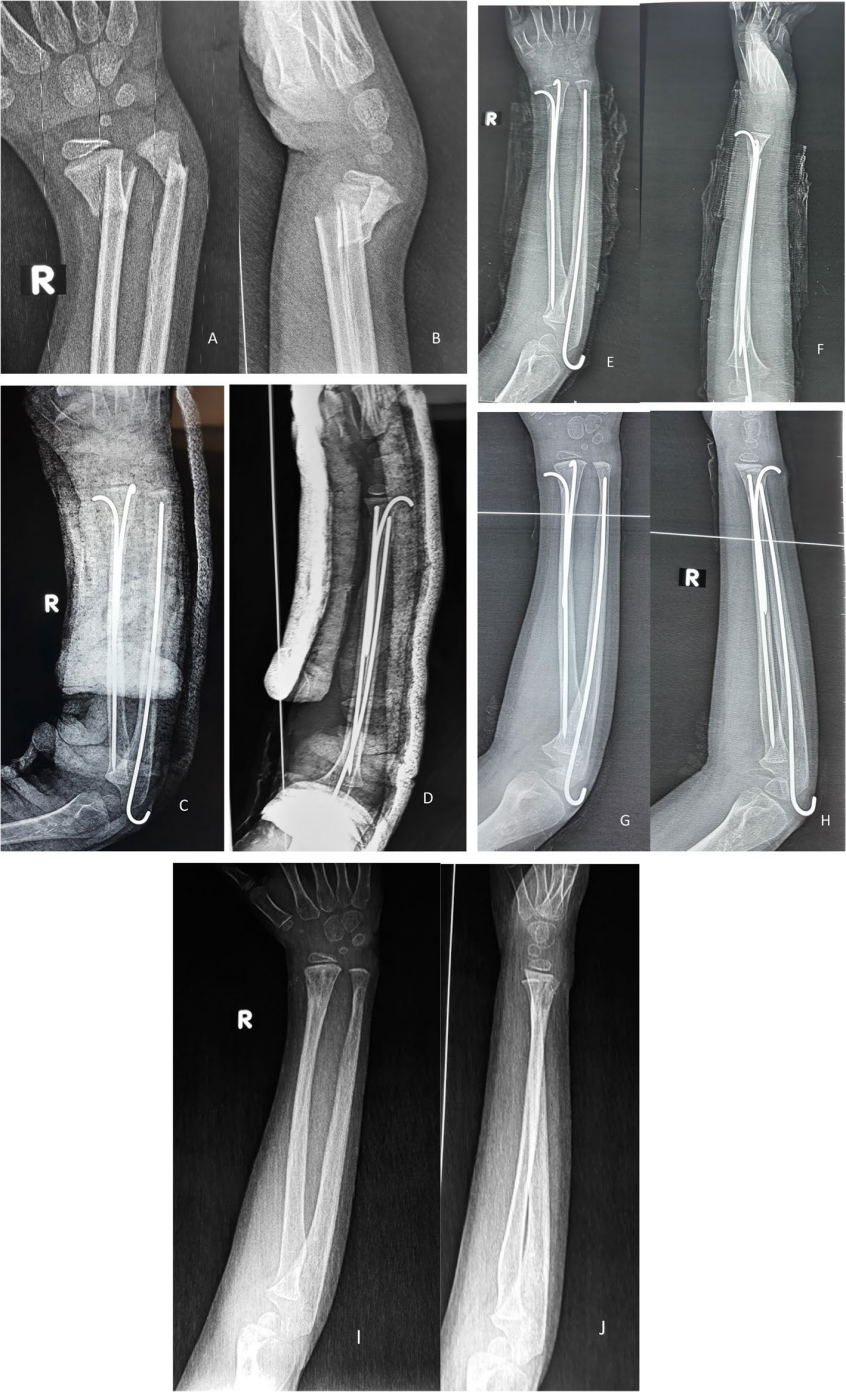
A and B. Anteroposterior and lateral X-rays show a distal forearm fracture in a male 7-year-old patient. C and D. Anteroposterior and lateral X-rays show the immediate postoperative radiograph. E and F. Anteroposterior and lateral X-rays show the follow-up radiographs 4 weeks postoperatively. G and H. Anteroposterior and lateral X-rays show the follow-up radiographs 8 weeks postoperatively with a complete union of both fractures. I and J. Anteroposterior and lateral X-rays show the follow-up radiographs at the end of follow-up after K-wire removal
